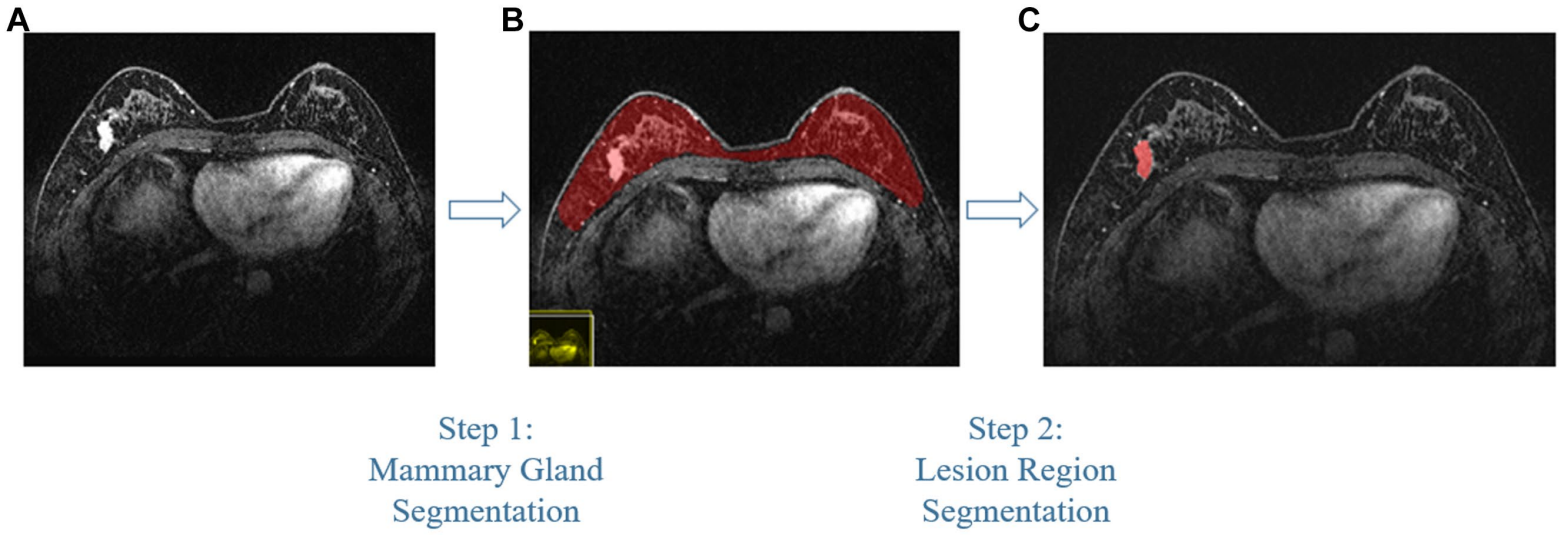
The proposed two-step breast cancer lesion segmentation method. (A) is a DCE-MRI image sample for breast cancer diagnosis, which contains irrelevant regions, such as the heart; (B) shows the segmented mammary gland region in red; (C) shows the breast cancer lesion region in red.
